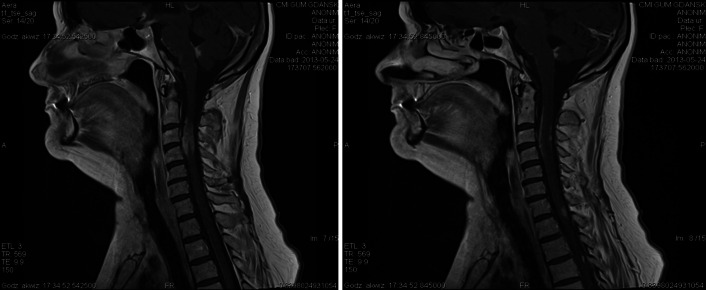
Neurosarcoidosis in MRI cervical spine in sagital plane: T1-weighted contrast-enhanced MR image shows enhancing leptomeningeal lesions involving of the spinal cord
